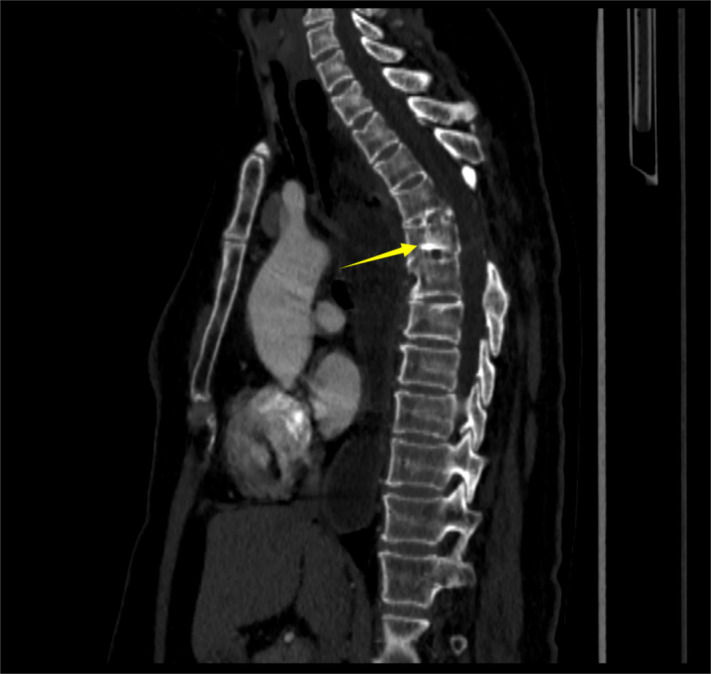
CT scan of the thoracic spine. Sagittal image at the T5–6 level shows heterogeneous density within the spinal canal (arrowhead), with disorganized bone structure and partial destruction of the vertebral bodies and posterior elements (arrow), consistent with chronic post-traumatic changes.
